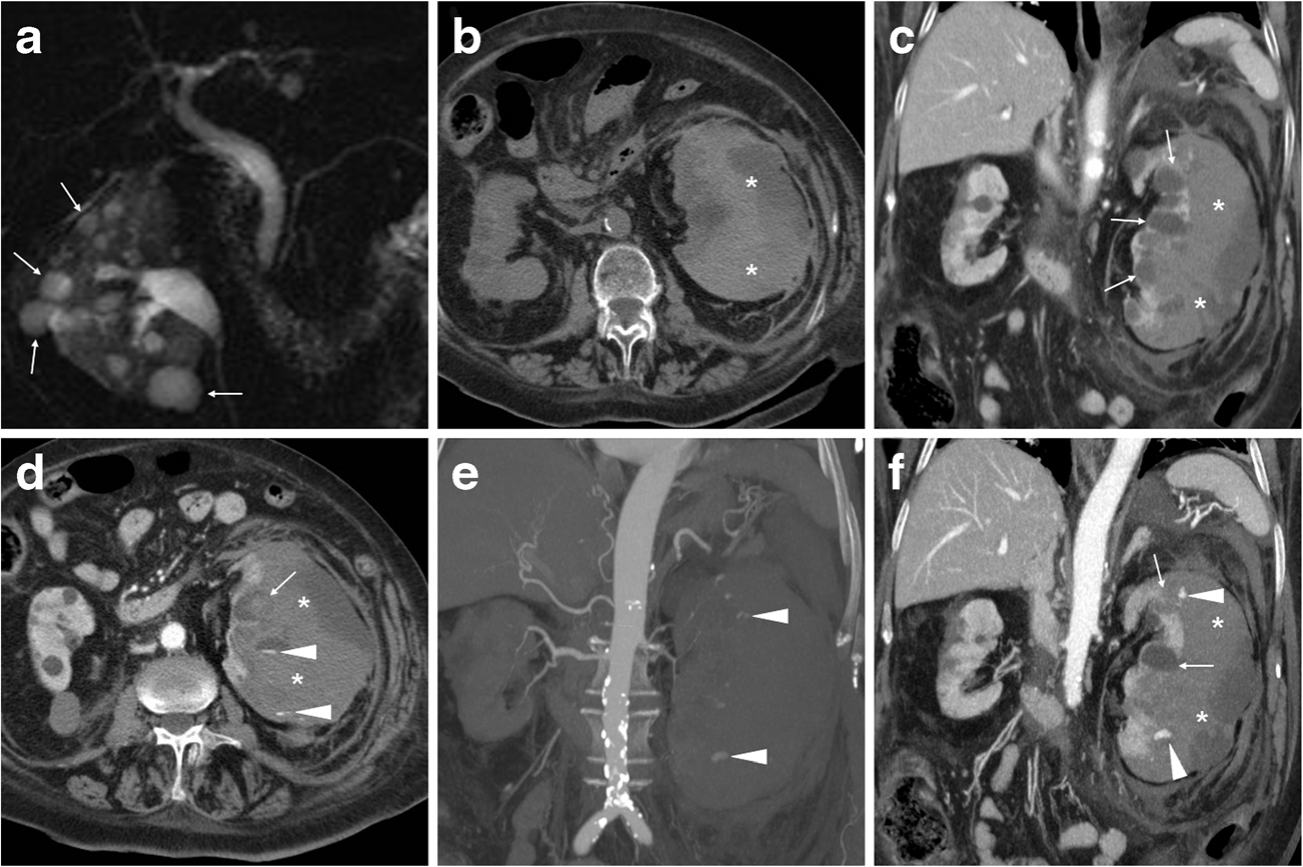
A 76-year-old female with several comorbidities, including hypertension, type II diabetes, epilepsy and chronic anaemia, suffered from severe pain and tenderness in her left lower abdomen. Features consistent with acquired cystic renal disese (ACKD) were noted in a magnetic resonance (MR)-cholangiopancreatography study (a) performed a few months earlier, including several moderately-sized cysts (*thin arrows*). Despite worsening end-stage renal disease (ESRD), she was not on haemodialysis and received antithrombotic prophylaxis after a previous deep venous thrombosis. Laboratory assays revealed a mild haemoglobin (8.2 g/dl) drop compared to baseline. At emergency department admission, an unenhanced multidetector CT study (b) was requested to investigate suspected acute diverticulitis. After detection of large left-sided subcapsular haematoma (*), a CT study was completed with contrast medium (CM) injection. Corticomedullary (c, d) and nephrographic (f) phase images showed the haematoma (*) exerting severe compression on the renal parenchyma, largely replaced by cysts (*thin arrows*) with mural discontinuity. Complemented with angiographic maximum-intensity projection (MIP) reconstructions (e), CT visualized small foci of CM extravasation isoattenuating with the blood pool (*arrowheads*). During renal arteriography (not shown), active bleeding was not observed anymore, indicating its spontaneous cessation. The patient slowly recovered during intensive care unit hospitalization, including blood transfusions and correction of metabolic acidosis
